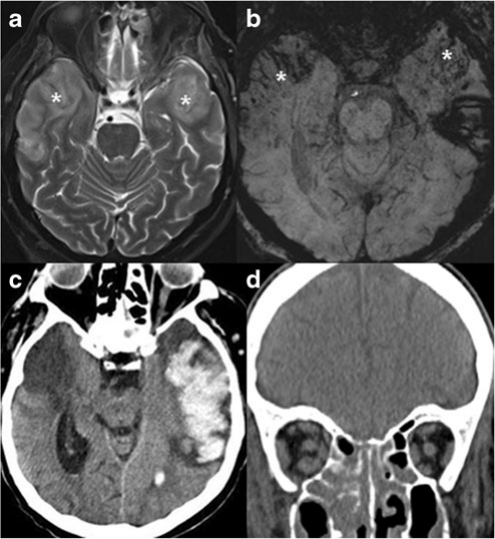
Mucormycosis infection of the temporal lobes bilaterally in a 62-year-old patient, immuncomprmised due to steroid treatment. Increased T2 signal (a) and blood products (b, SWI) are noted in the anterolateral temporal lobes (*asterisks*). The patient later developed massive left temporal lobar haemorrhages (c), and deceased. On coronal CT image of the same patient (d), note partial opacification of the ethmoid and maxillary sinuses and stranding of the intra-orbital fat. Autopsy revealed mucormycosis in brain parenchyma and paranasal sinuses
